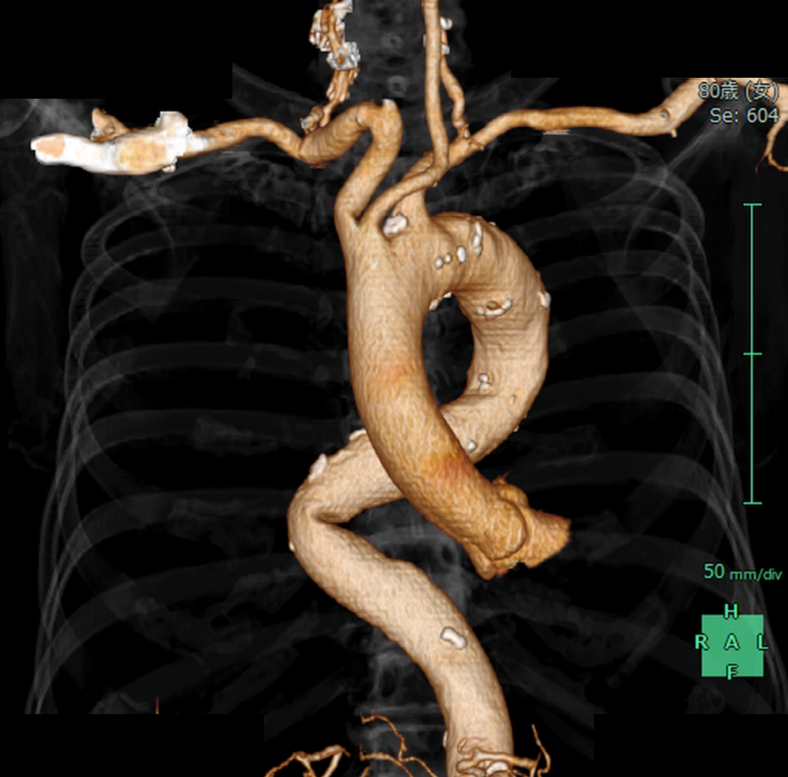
Three-dimensional reconstructed CT demonstrating marked tortuosity of the descending thoracic aorta. The descending thoracic aorta courses behind the left atrium and is in close proximity to the esophagus, suggesting a potential site of chronic mechanical compression.
